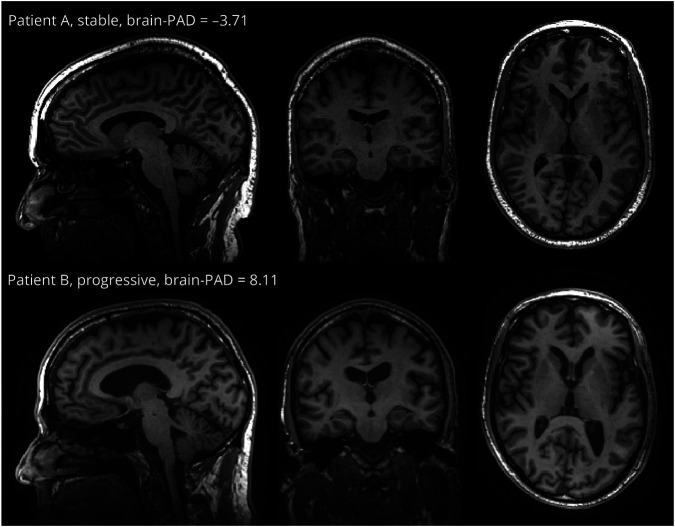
MRI-Scans From Converting and Nonconverting Patients With SCD Matched on MRI-Scanner, Age, Sex, MMSE, and Visual Atrophy Scale Scores brain-PAD = brainpredicted age difference; MMSE = Mini Mental State Examination; SCD = subjective cognitive decline.
